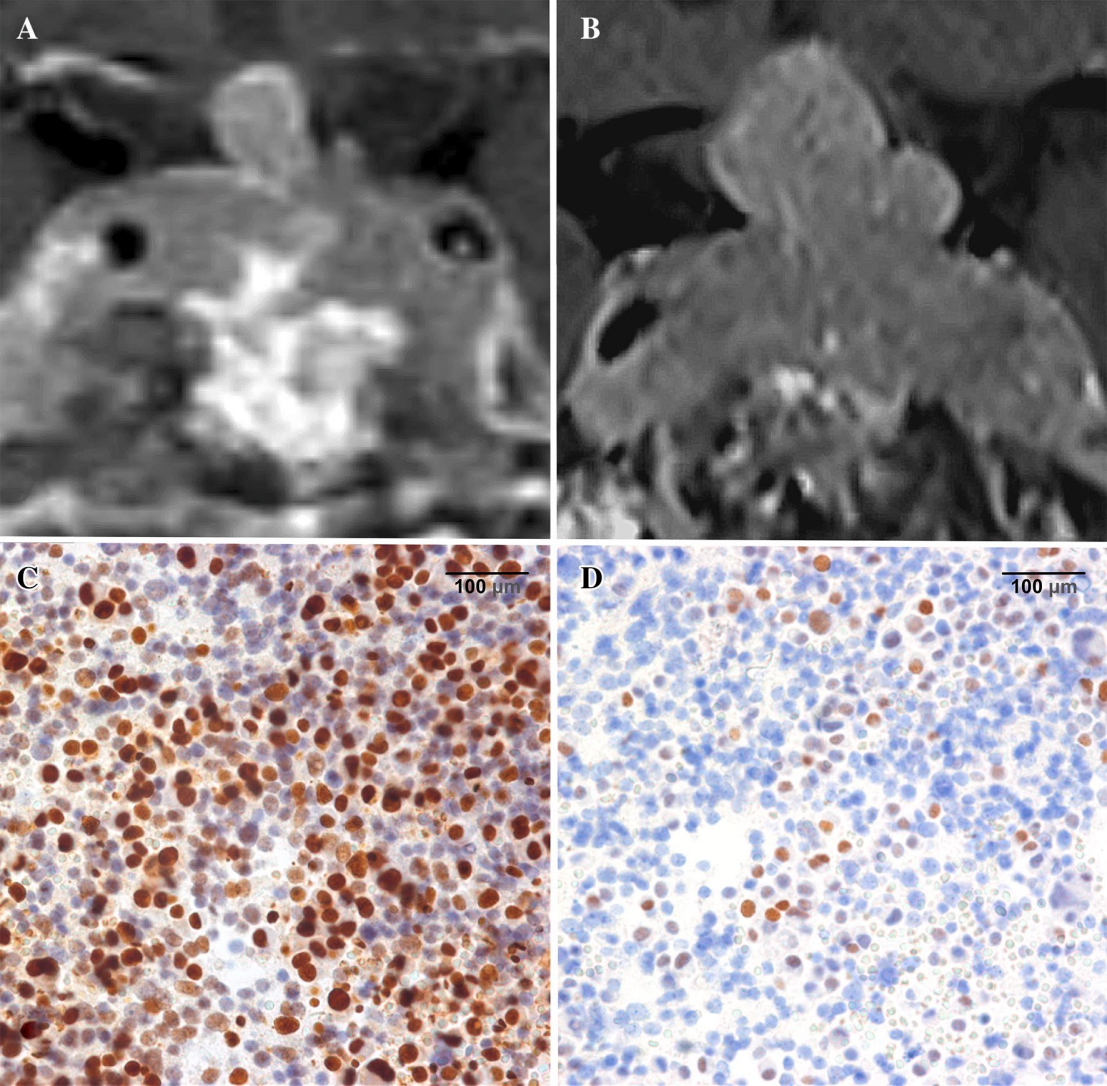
TMZ non-responder—Nelson tumor in a 55-year-old female patient. a Coronal MRI at the start of TMZ treatment shows recurrence of the invasive adenoma with high MIB-1 (13.8%) despite multimodal treatment (multiple transsphenoidal operations, 54 Gy external radiation, pasireotide therapy). b Coronal MRI 6 months after the first TMZ cycle; tumor regrowth occurred under therapy. Due to visual decline, TMZ was discontinued after 4 months and microsurgical partial resection of the tumor recurrence was performed by a subfrontal approach. c MGMT IHC sample from the last operation before TMZ treatment shows high MGMT immunoexpression (>50%) ×40 magnification. d MSH6 IHC sample from the last operation before TMZ treatment shows high MSH6 immunoexpression (10–50%) ×40 magnification
